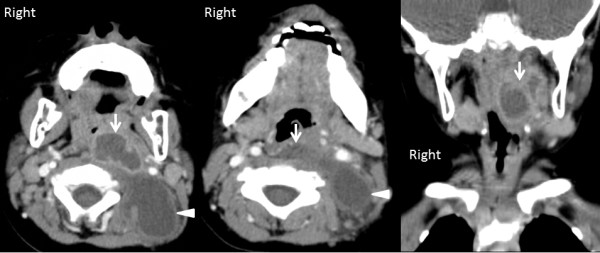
Neck computed tomography angiography findings. There was a low-density cavity surrounded by a high-density area (arrow) in the retropharyngeal space. A poorly enhanced low-density area was also observed in the posterior neck (arrowhead).
